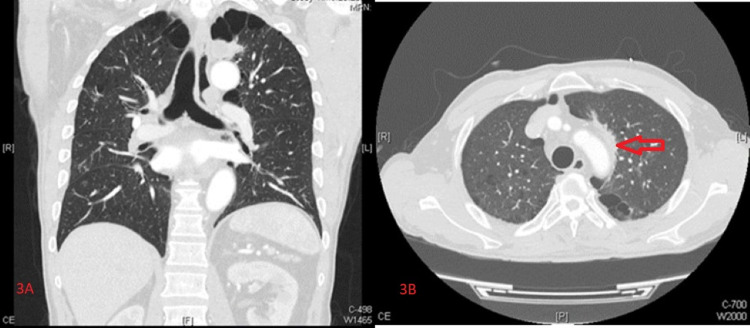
Chest computed tomography with iodine contrast. Coronal and axial images showing a reduction in the size of the left apical mass after antifungal therapy.
